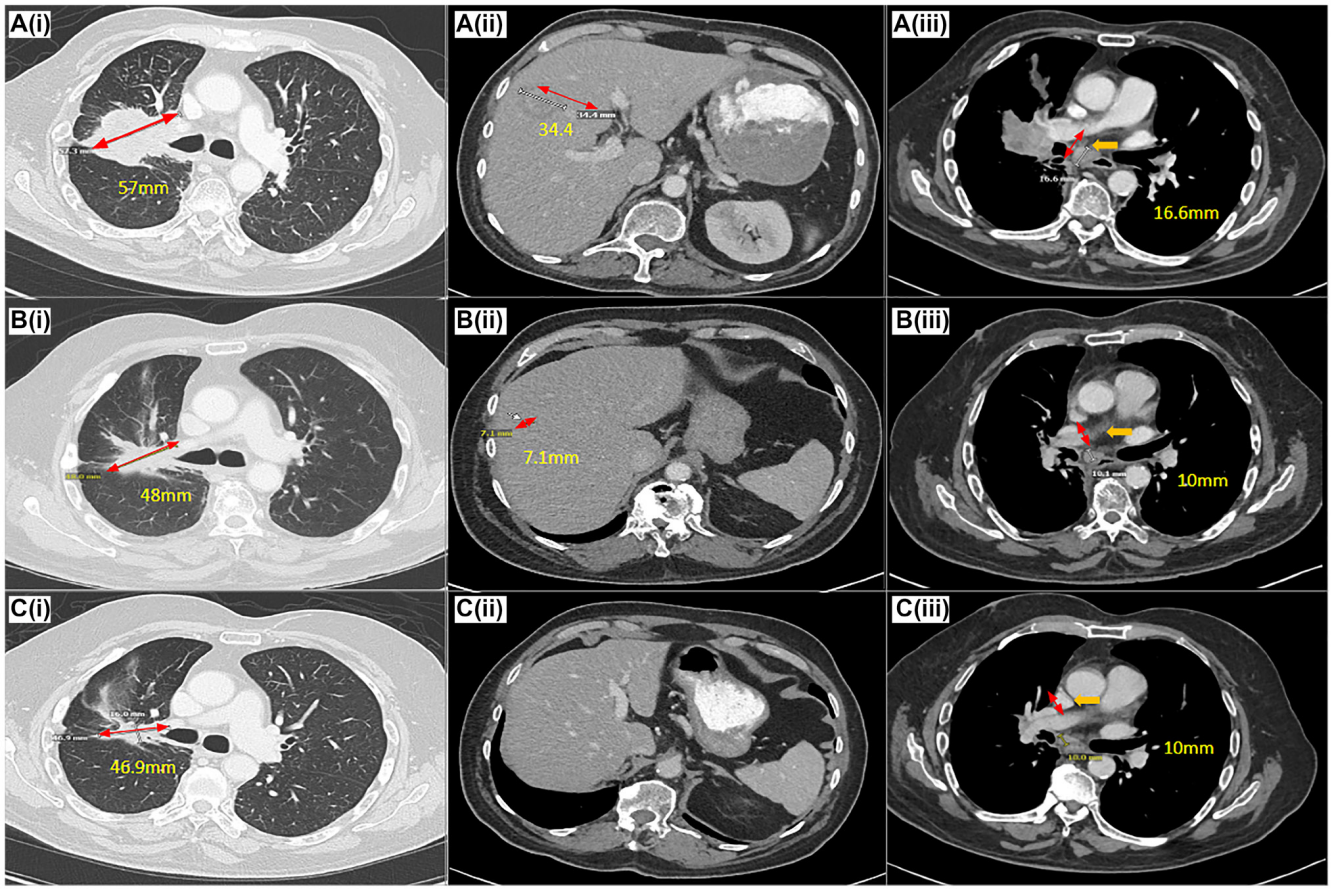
Target response on amivantamab based on RECIST 1.1 on CT scan. CT Chest (i), Abdomen/Pelvis (ii)(iii) at the time of pre-treatment (A), 6 months (B) (complete resolution of CNS disease), 19 months (C) demonstrating a decrease in size of the patient’s lung, liver, mediastinal lesions after treatment with amivantamab. Pre-treatment CT chest with a right upper lobe/perihilar mass, measuring 57 mm in the long axis (A(i)), decreased to 48 mm (B(i)) and 46.9 mm (C(i)) on post-treatment scans. Pre-treatment CT abdomen with a lobular liver lesion, measuring 34.4 mm in the long axis (A(ii)), decreased to 7.1 mm (B(ii)) and complete resolution (C(iii)) on post-treatment scans.
